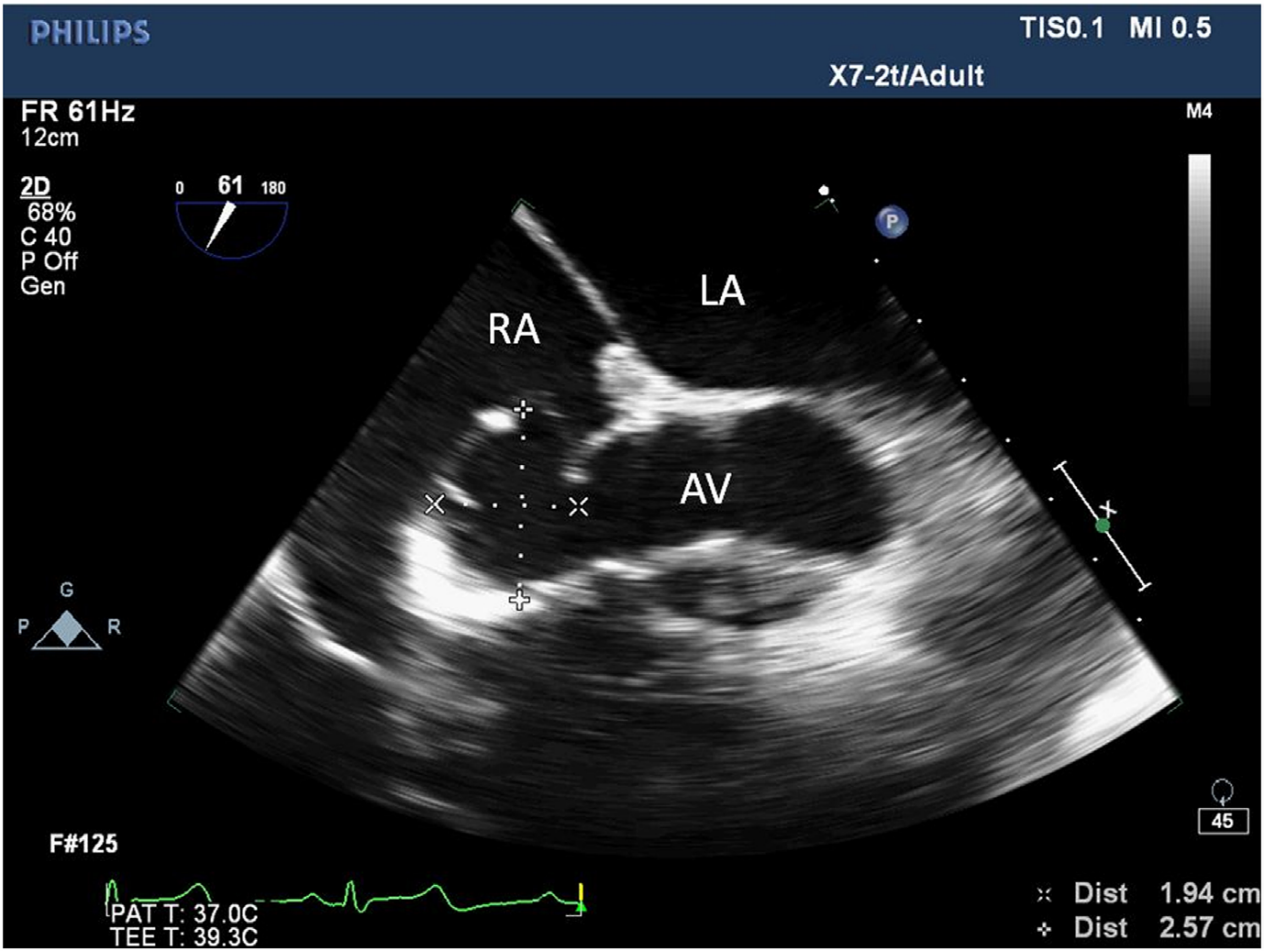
Two-dimensional echocardiogram short-axis view of the aortic valve showing an aneurysm at the non-coronary sinus of Valsalva measuring 1.94 cm × 2.57 cm. LA, left atrium; RA, right atrium; AV, aortic valve.
